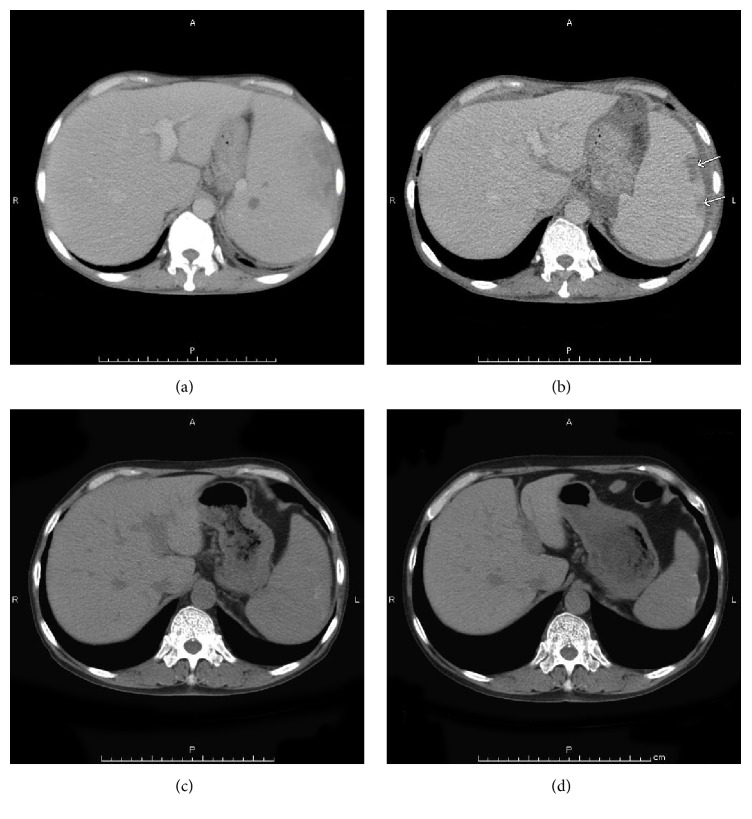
Case  1.* Computed tomography (CT). (a) Before HCT, the CT showed severe splenomegaly. (b, c, d) After HCT, the massive splenomegaly was gradually reduced. (b) Three months. (c) Six months. (d) Twelve months. The arrow shows infarction.
